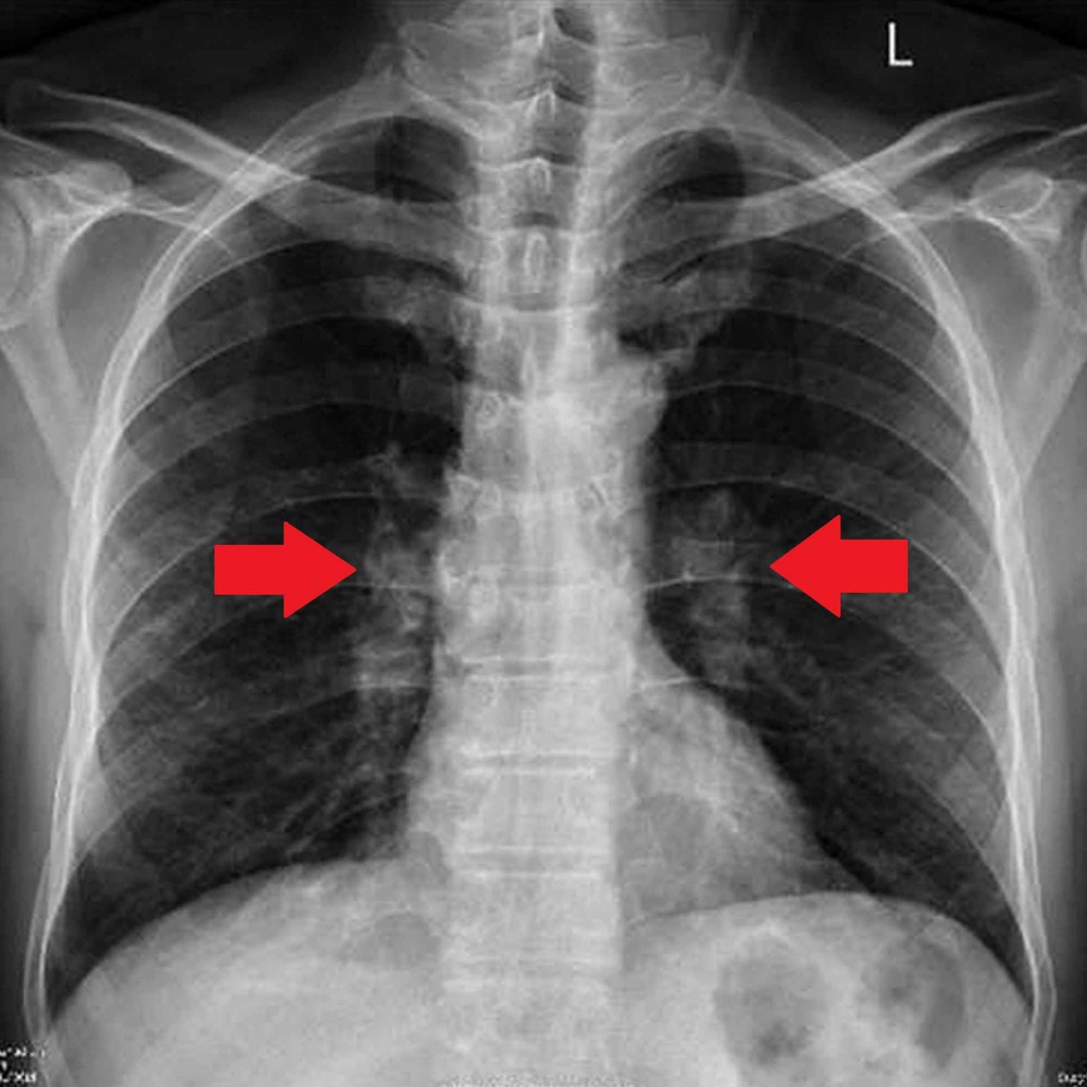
Chest x-ray before treatment with doxycycline with presence of bilateral hilar lymphadenopathy (red arrows).
